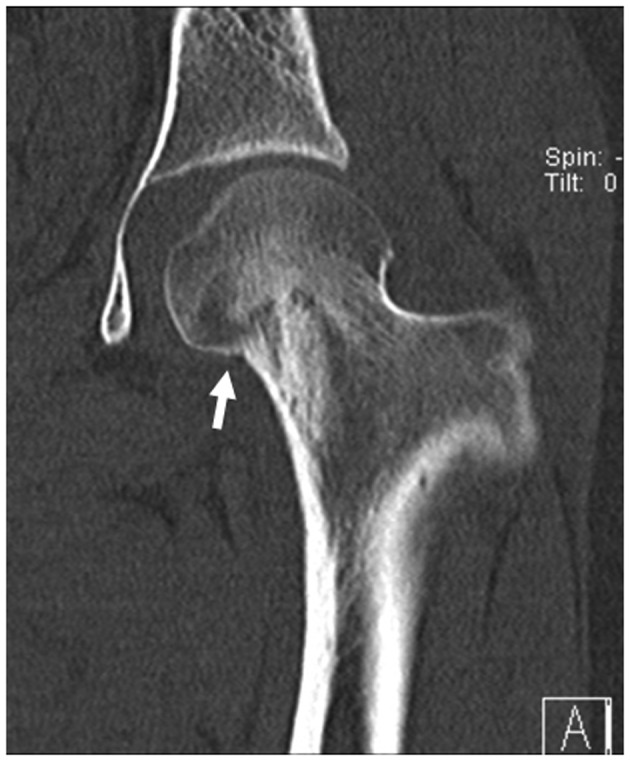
Computed tomography (CT) observations at the time of the initial consultation showing discontinuity of the cortical bone at the medial side of femoral neck (arrow). A periosteal reaction is not shown..
